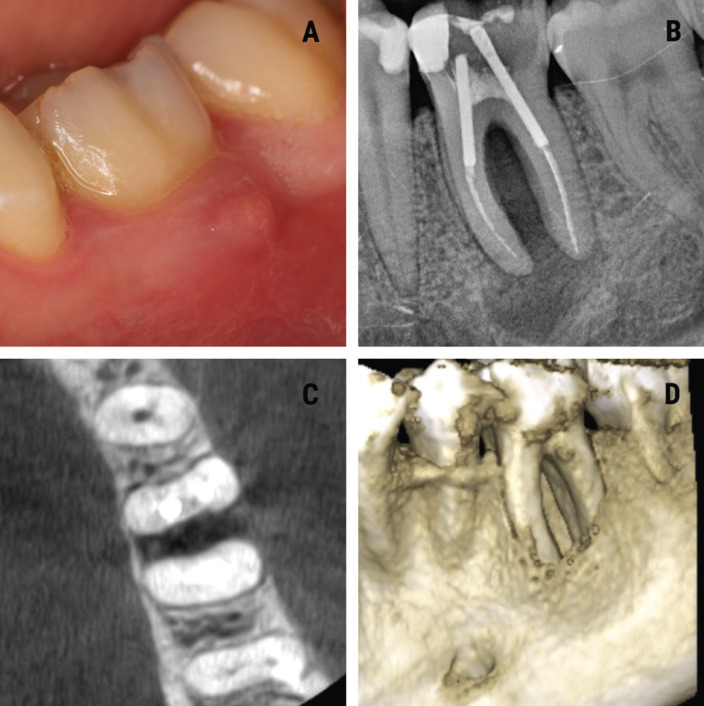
A. Intraoral view showing an abscess in the attached gingiva at level 3.6. B. Periapical radiograph showing a radiolucency along the roots of 3.6. C. Tomographic (CBCT) axial sectional view where destruction of the vestibular cortical layer and involvement of the bone around the furrow of 3.6 can be seen. D. Three-dimensional tomographic (CBCT) reconstruction showing the bone lesion around the entire root of tooth 3.6.
